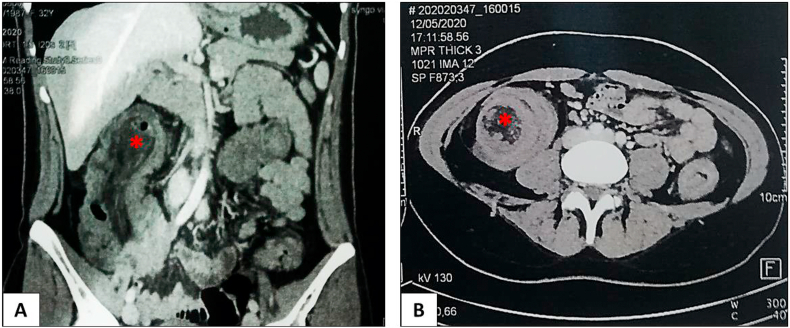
(A): CT of abdomen showed ileocolic intussusception (asterisks), which extended over 18cm with “target sign” (B).
